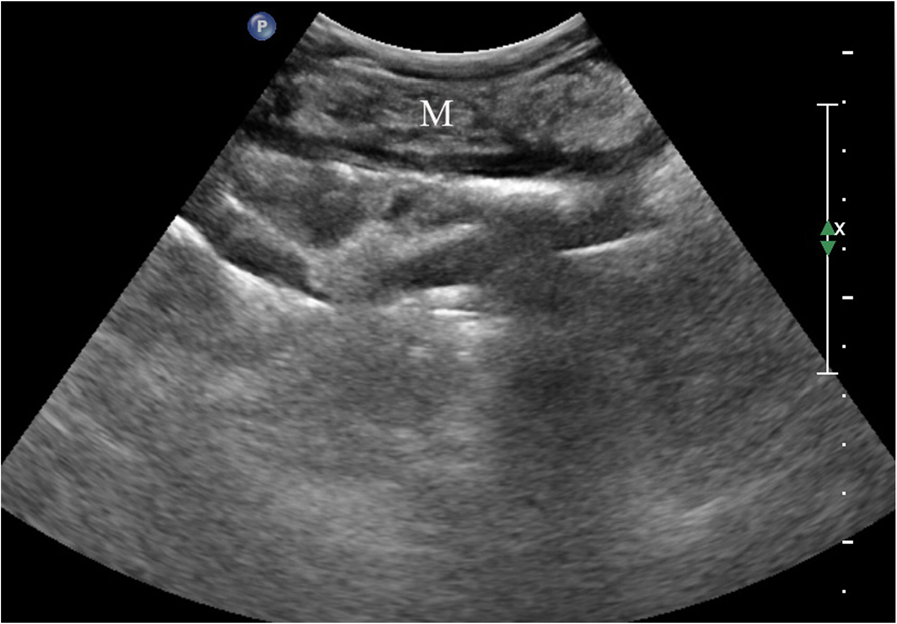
Data for a 37-year-old man. Ultrasonography showed a cake-shaped thickening of the omentum (M) with homogeneous hyperechoes; this thickening was then pathologically diagnosed as tuberculosis of the peritoneum.
